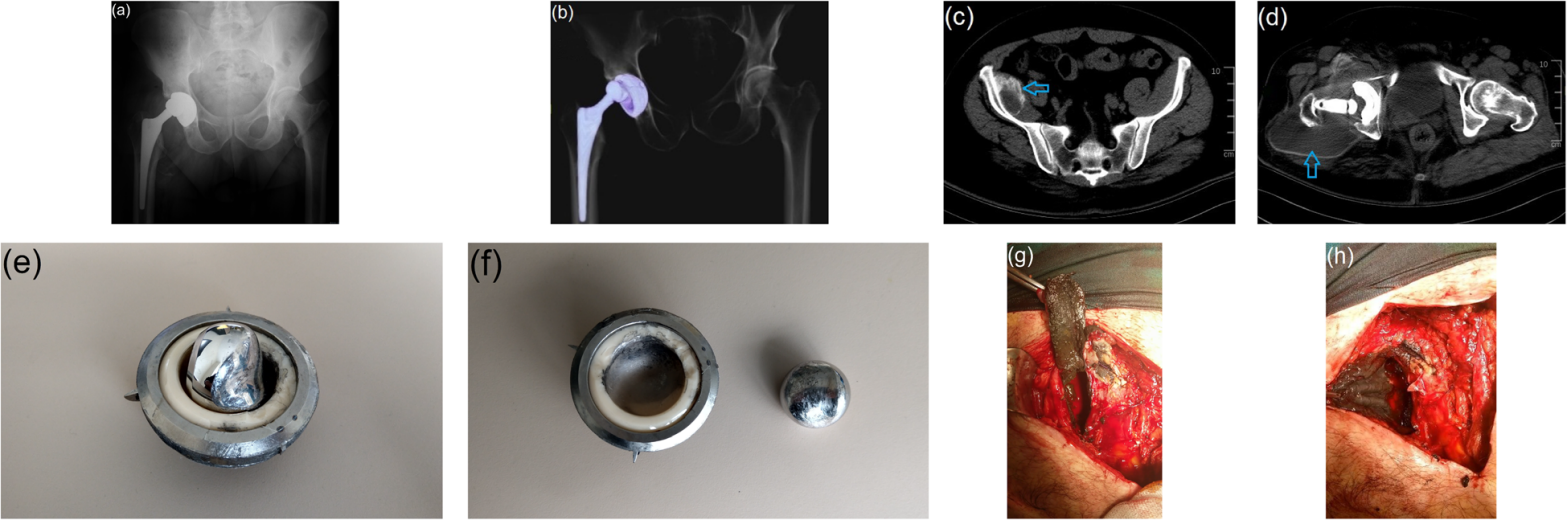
a, b Anteroposterior radiographic and 3D computed tomographic (CT) images of catastrophic acetabular component wear in a 49-year-old man with primary total hip arthroplasty with metal-on-ceramic bearing, 3 years postoperatively. c, d Axial CT images of the high-density pseudocystic structure extending into the pelvis in the right hip and surrounding the thigh widely (arrow). e, f View of deformation of the acetabular components. g, h Intraoperative appearance of diffuse metallosis developing in periprosthetic tissues
